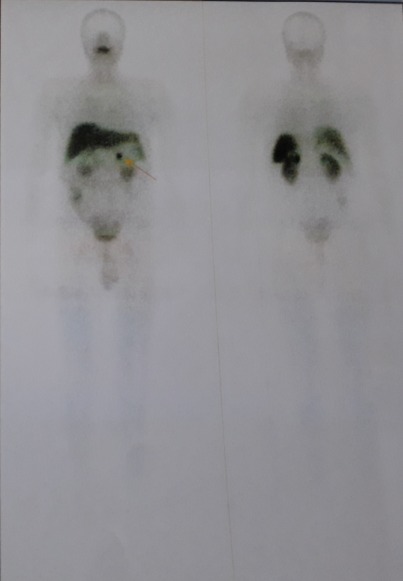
Image scintigraphique d'une tumeur endocrine du pancréas
